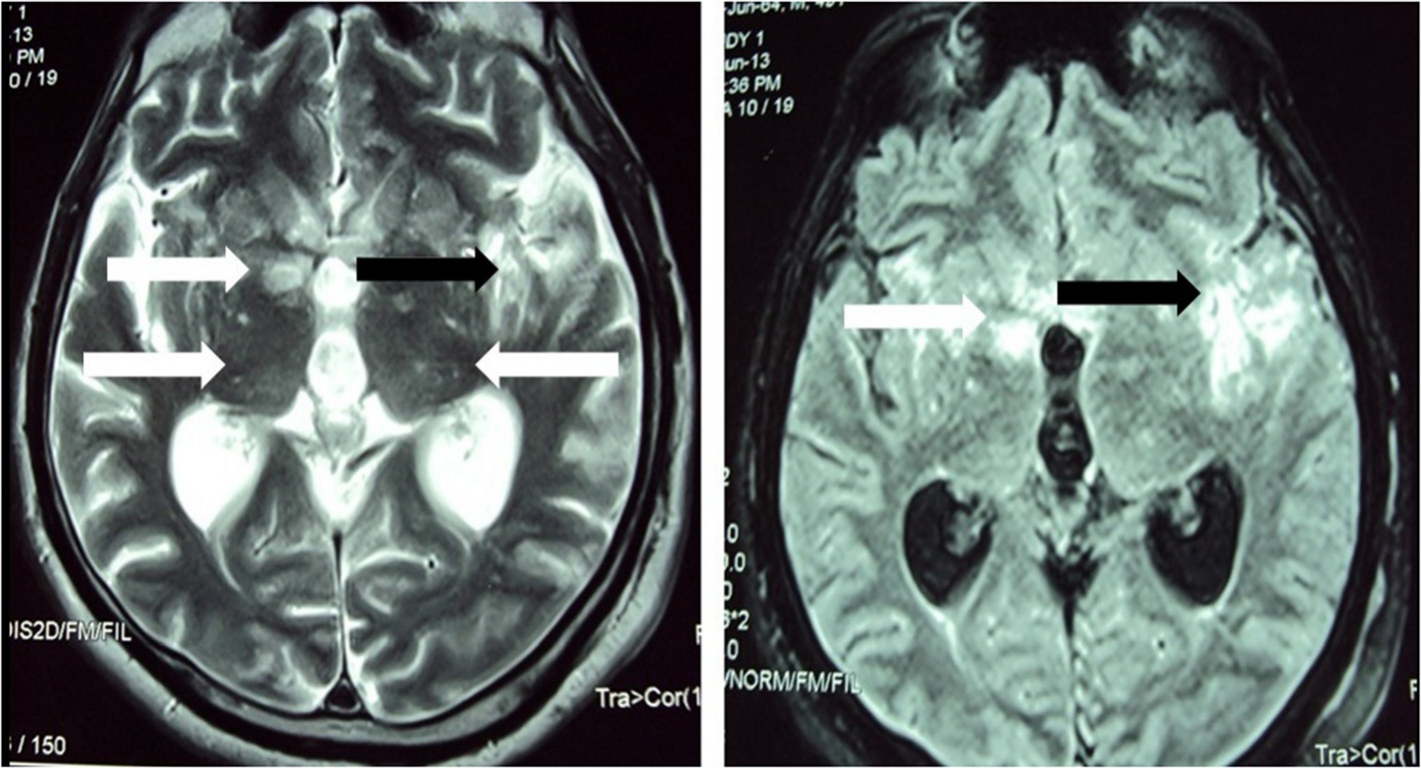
Fluid attenuated inversion recovery (FLAIR) (Right) and T2-weighted (Left) images of magnetic resonance imaging (MRI) of brain. Bilateral multiple nodular lesions in basal ganglia and thalamus (white arrows). Ill defined area of signal intensity change in the left temporoparietal region (black arrows).
